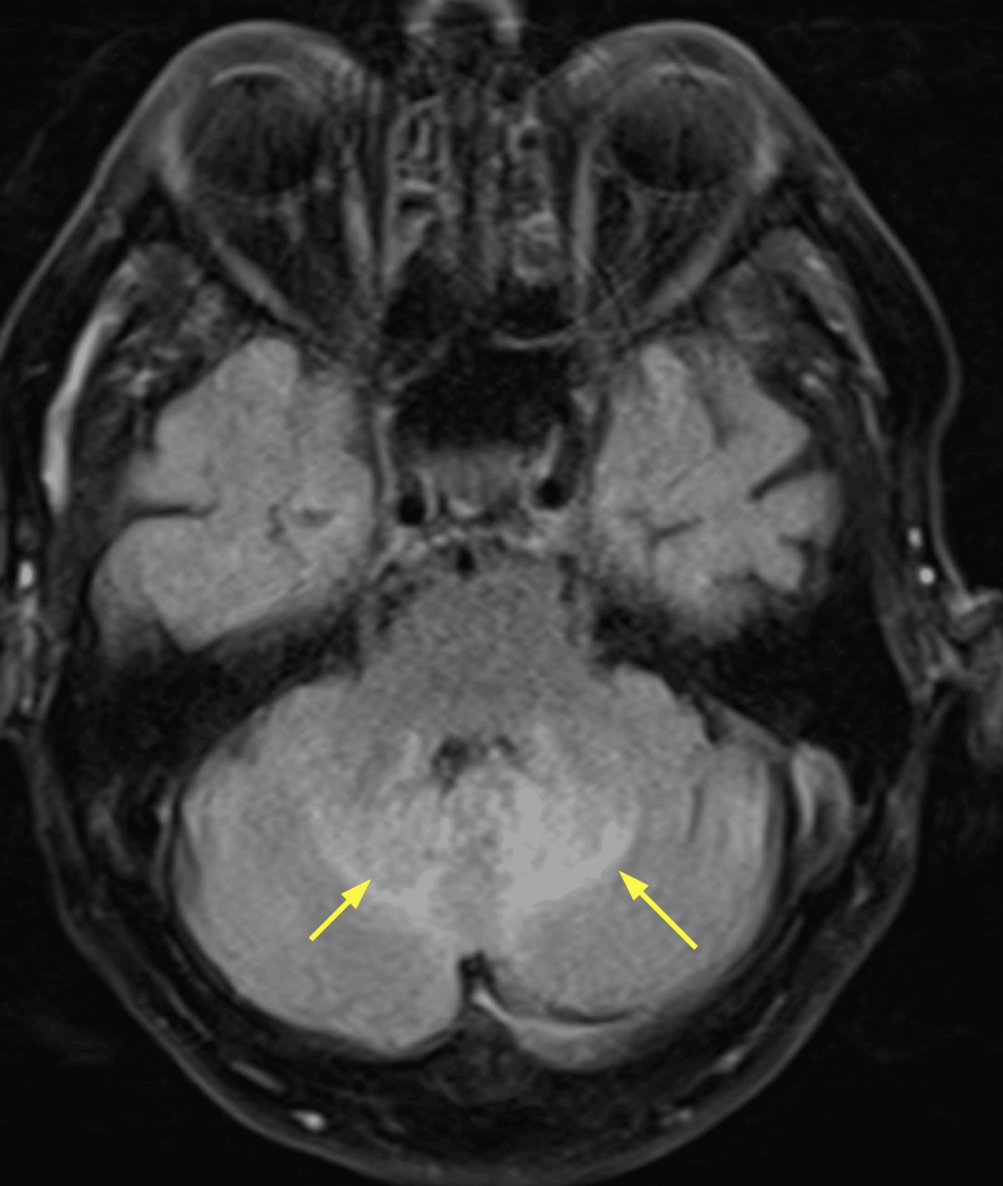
Repeat MRI brain on day 15 of admission: cerebellar section Ongoing changes within the cerebellum were noted, with high FLAIR signal persisting within the folia. There remains a distortion of the fourth ventricle. Two yellow arrows are pointing to these bilateral cerebellar lesions. FLAIR, fluid-attenuated inversion recovery
